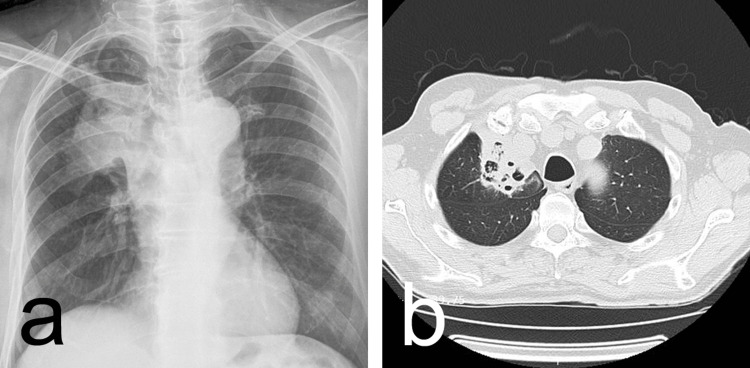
(a) An X-ray revealing diffuse opacity indicative of an infiltrative process in the medial aspect of the right lung's upper lobe, just above the hilum; (b) Computed tomography revealing a diffuse infiltrative process in the medial portion of the right upper lobe, with pleural involvement.
